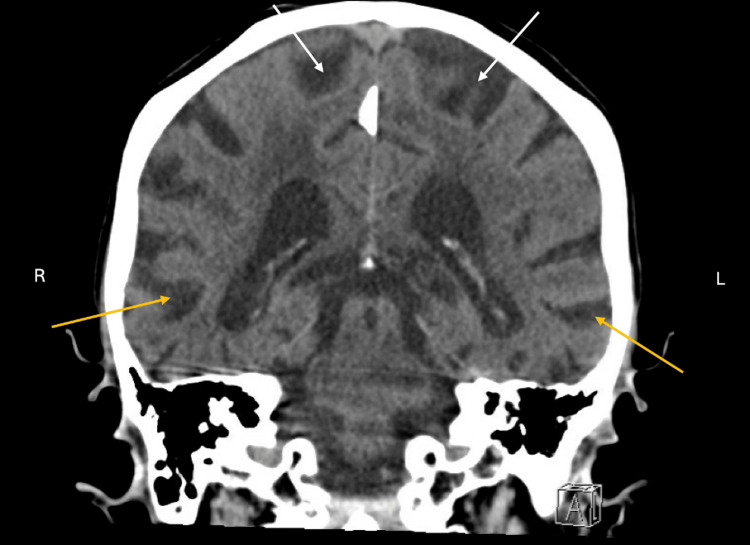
CT brain without contrast, coronal view. Diffuse cerebral volume loss was noted (white arrows) with more loss in the right temporal lobe compared to the left (yellow arrows).
